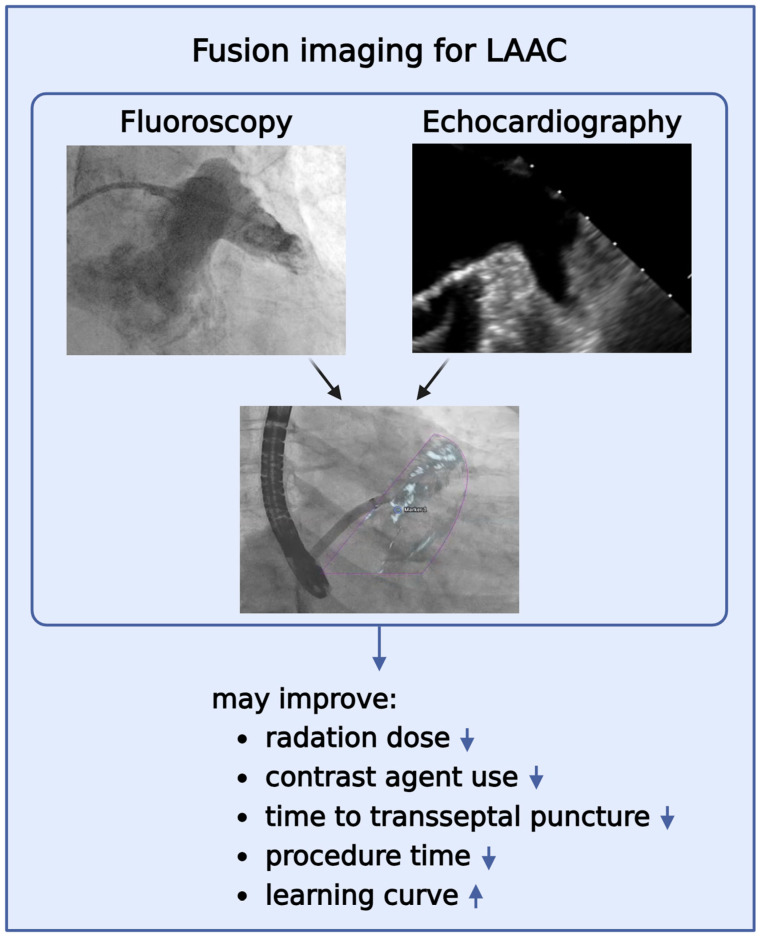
Benefits of FI for LAAC. It may lead to a reduction in radiation dose, contrast agent use, time to transseptal puncture, procedure time, and may optimize the learning curve. Purple triangle depict real-time fluoroscopic and echocardiographic overlay.
